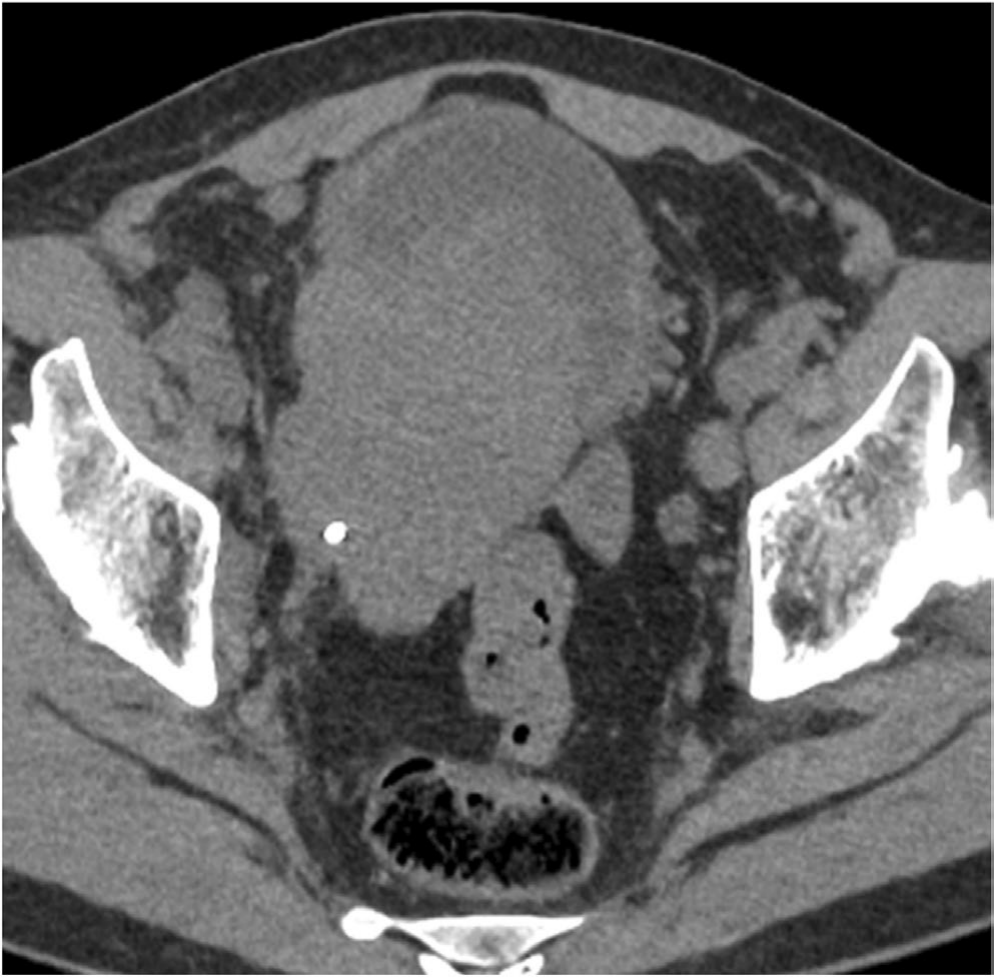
The chest and abdominal CT findings indicate that the bladder tumor has significantly increased in size, with evidence of bilateral hydronephrosis and a metastasis to the right rib.
